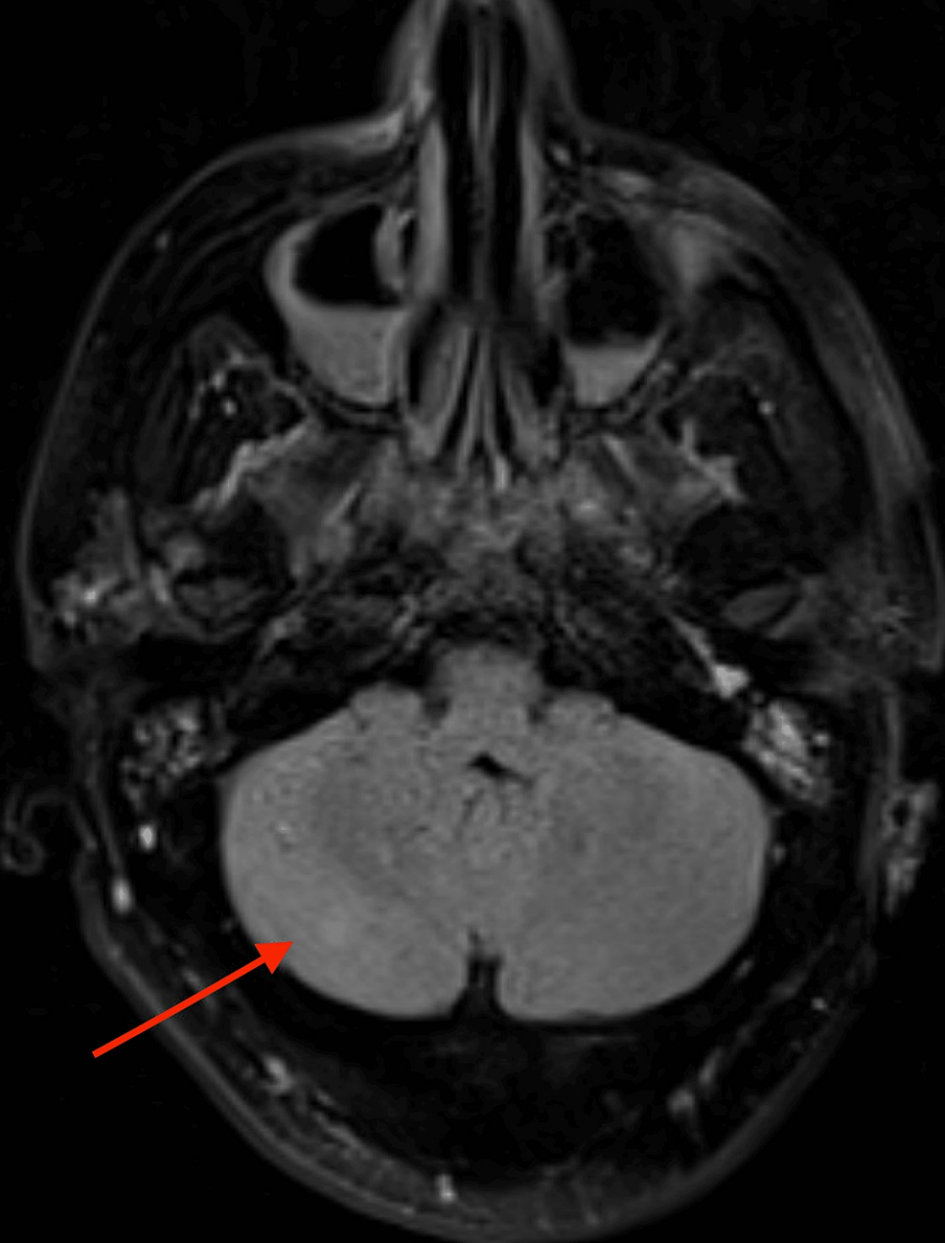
The same fluid-attenuated inversion recovery magnetic resonance imaging (FLAIR MRI) showing an infratentorial lesion in the right cerebellar hemisphere (red arrow).
